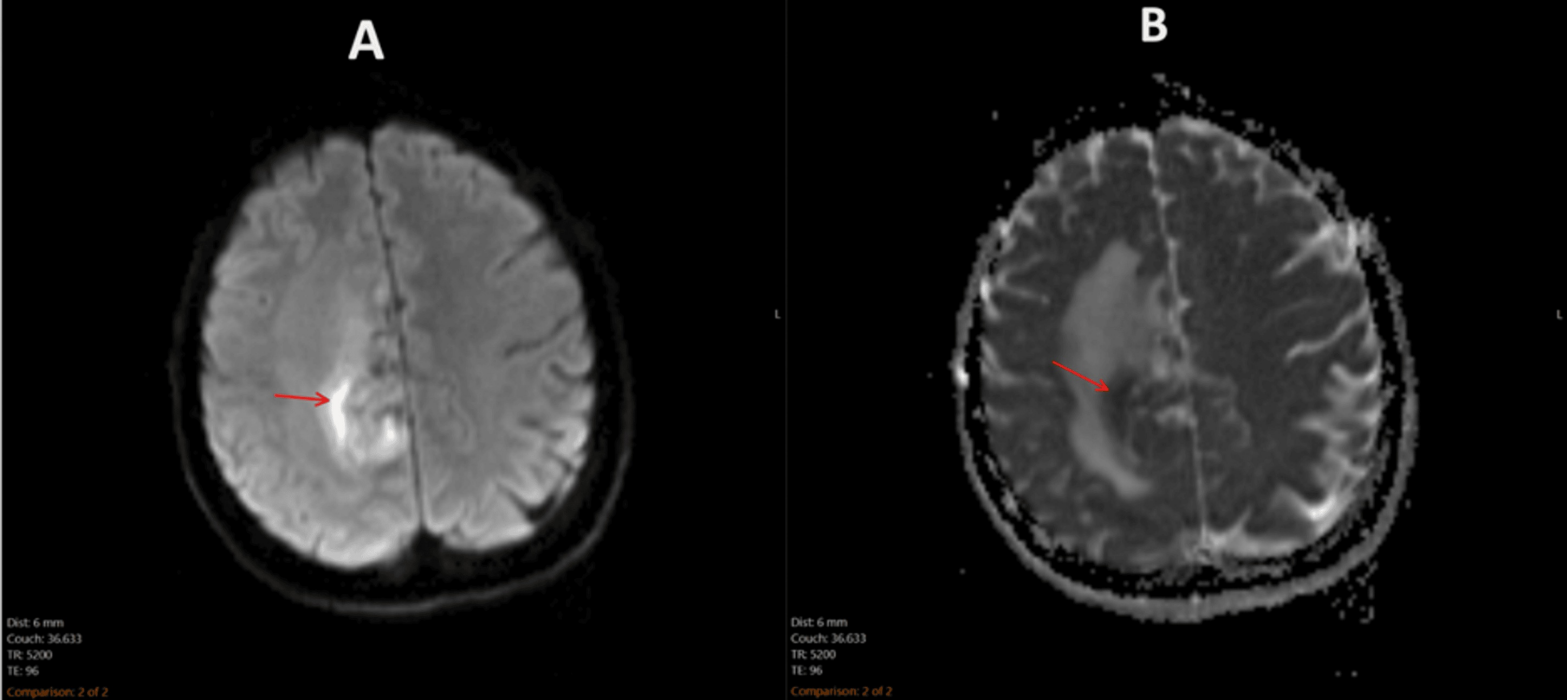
DWI and corresponding ADC mapping of a right frontoparietal lesion (A) DWI demonstrates an intense signal at the right frontoparietal lesion (arrow). (B) Corresponding ADC mapping confirms the presence of true diffusion restriction (arrow). DWI: diffusion-weighted imaging; ADC: apparent diffusion coefficient
